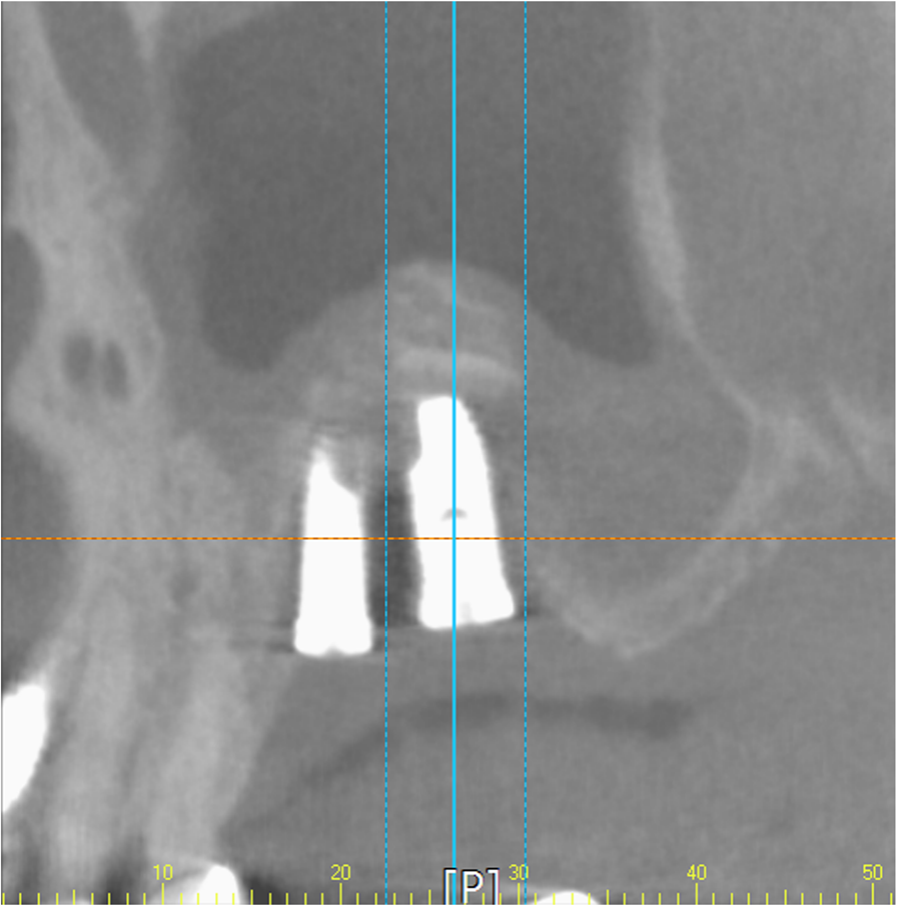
Postoperative CBCT view 4 months later
